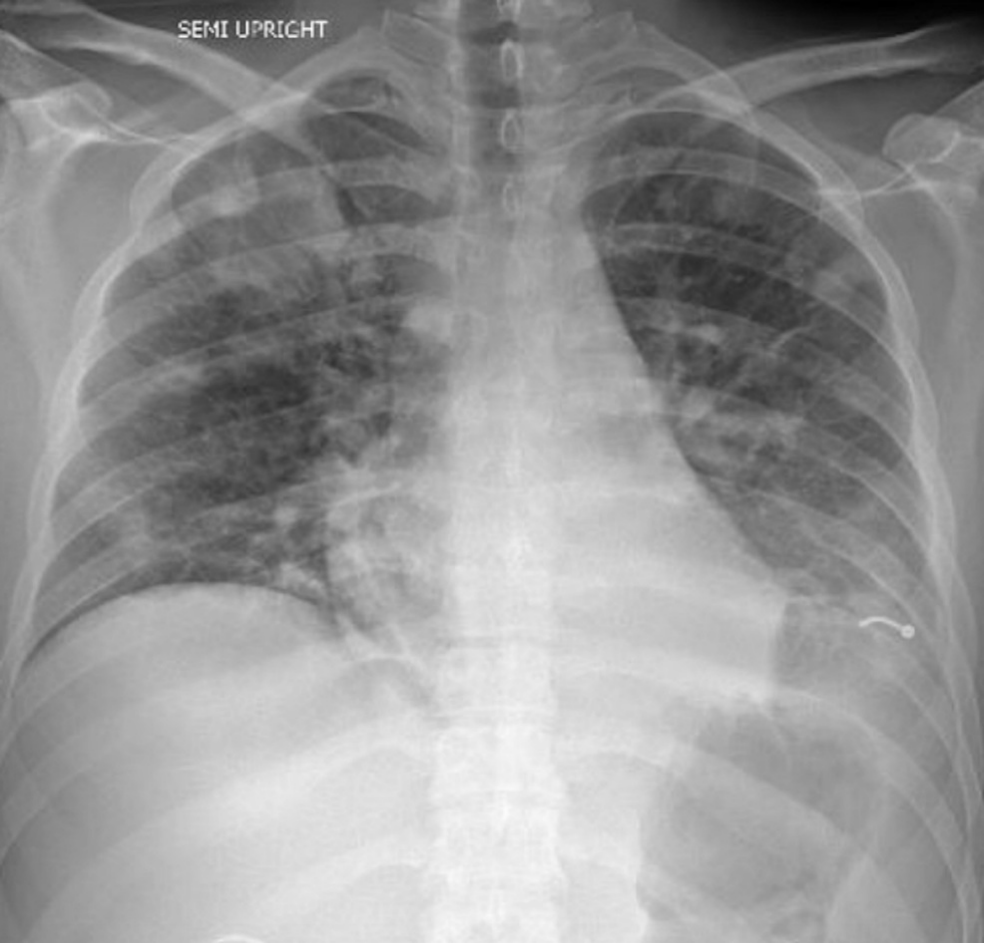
Bilateral diffuse nodular infiltrates on chest x-ray. Artifact noted at the left pulmonary base.
